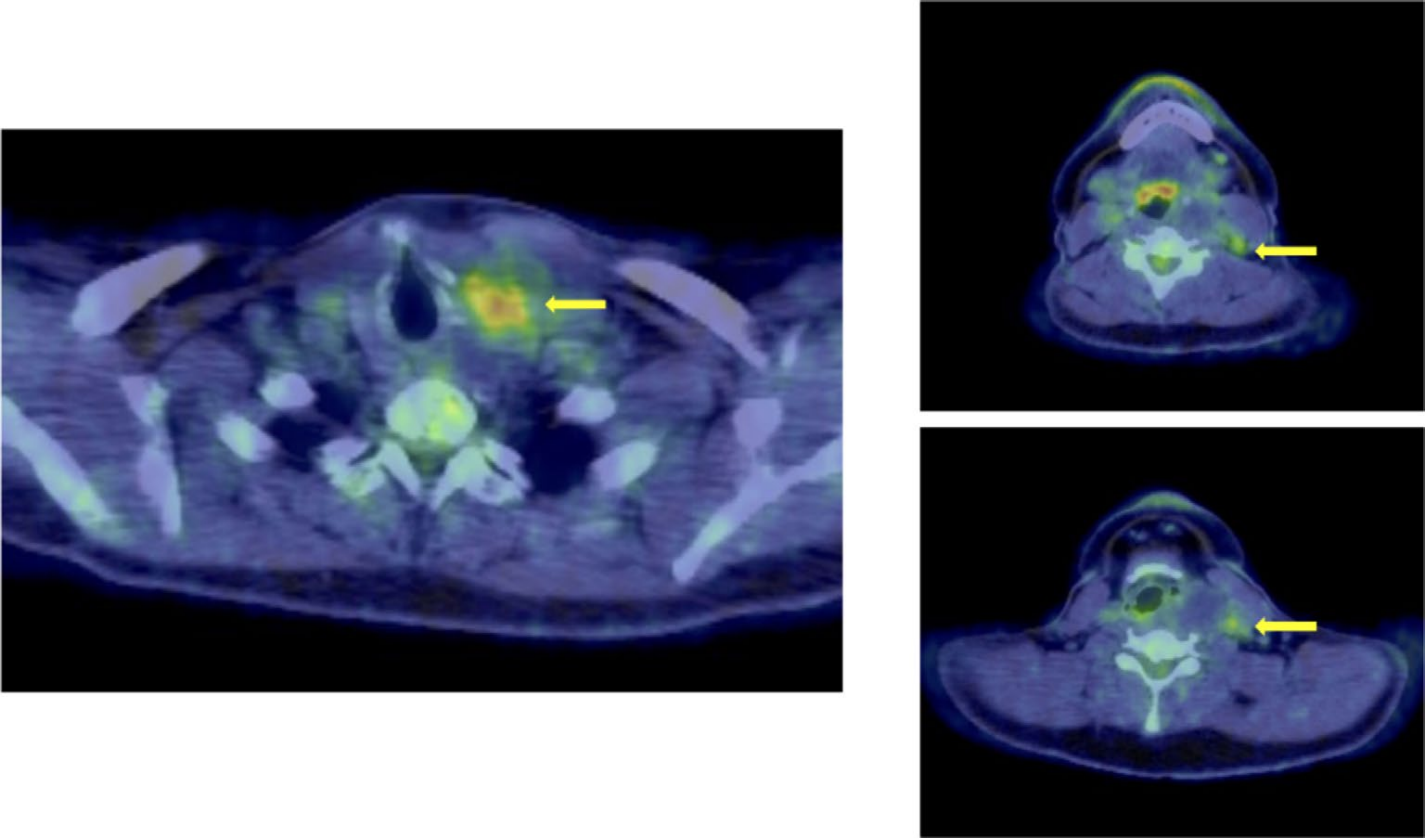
PET/CT images. Fluorodeoxyglucose (FDG) accumulation was observed in the left lobe of the thyroid gland and left cervical lymph nodes. The yellow arrow indicates FDG accumulation.
